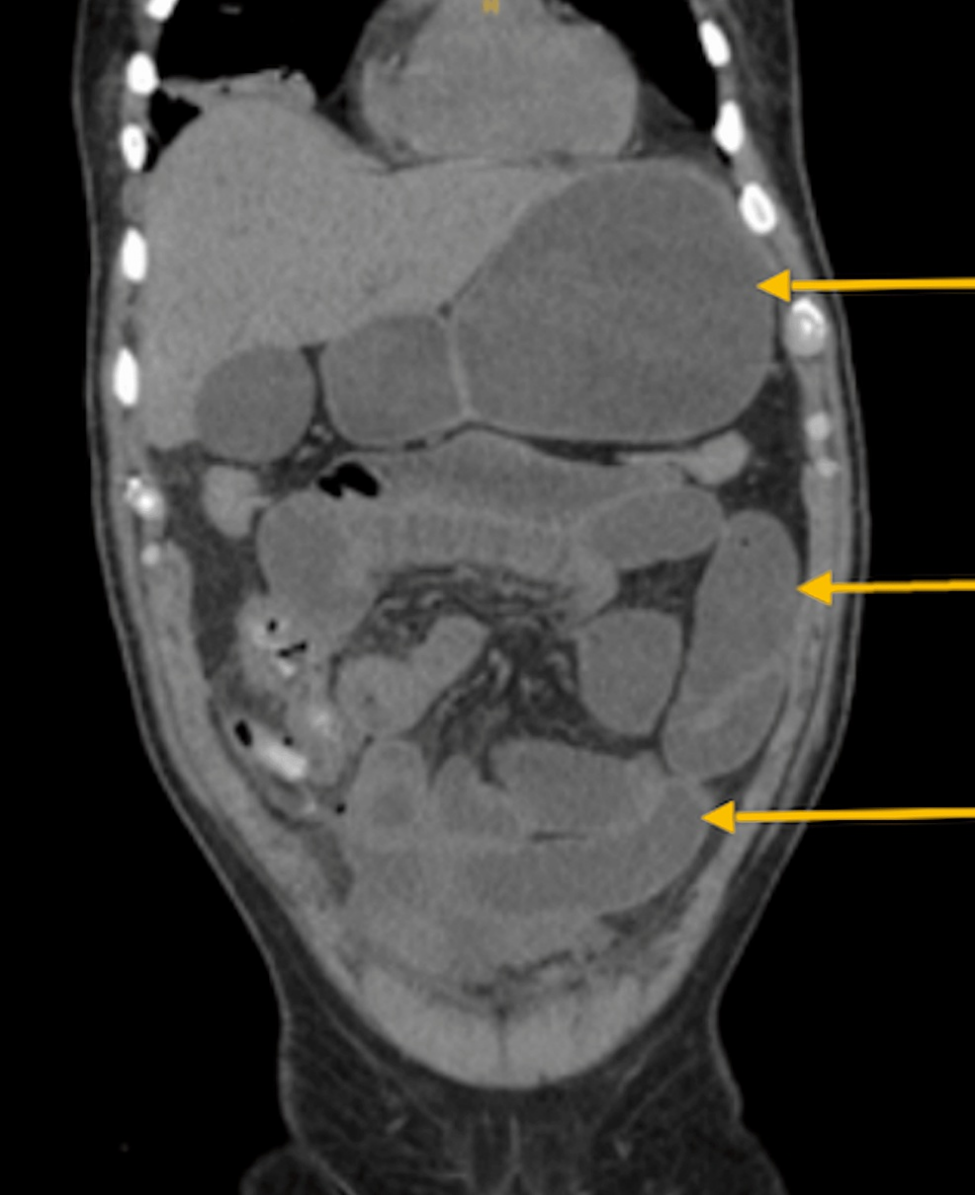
CT obtained on ED presentation, showing evidence of SBO with dilated loops of bowel (yellow arrows) SBO: small bowel obstruction
